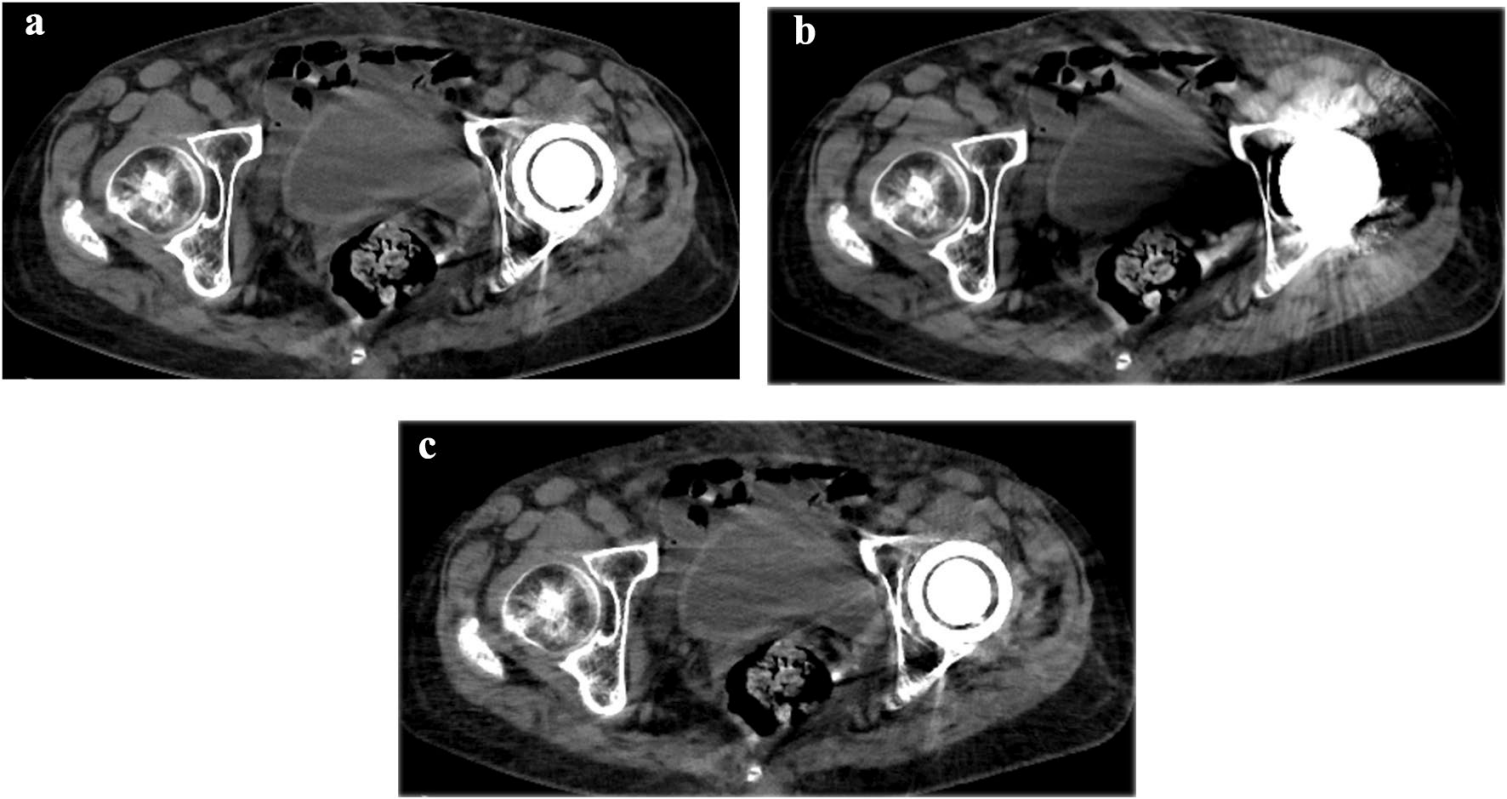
Axial unenhanced CT images reconstructed with DLR-S (a), DLR (b), and IR-S (c) of an 84-year-old woman. The degree of metal artifacts (DLR-S/DLR/IR-S) was rated 4 (minimal artifacts)/1 (severe artifacts in a large area)/2 (severe artifacts in a small area) by reader 1. It was rated 3 (moderate artifacts)/2 (severe artifacts in a small area)/3 (moderate artifacts) by reader 2. Bladder delineation (DLR-S/DLR/IR-S) was rated 3 (moderately blurred)/1 (unrecognizable)/2 (notably blurred) by reader 1. Reader 2 rated it as 2 (noticeably blurred)/2 (noticeably blurred)/3 (moderately blurred). No lesions were noted in the artifact slice in this case
